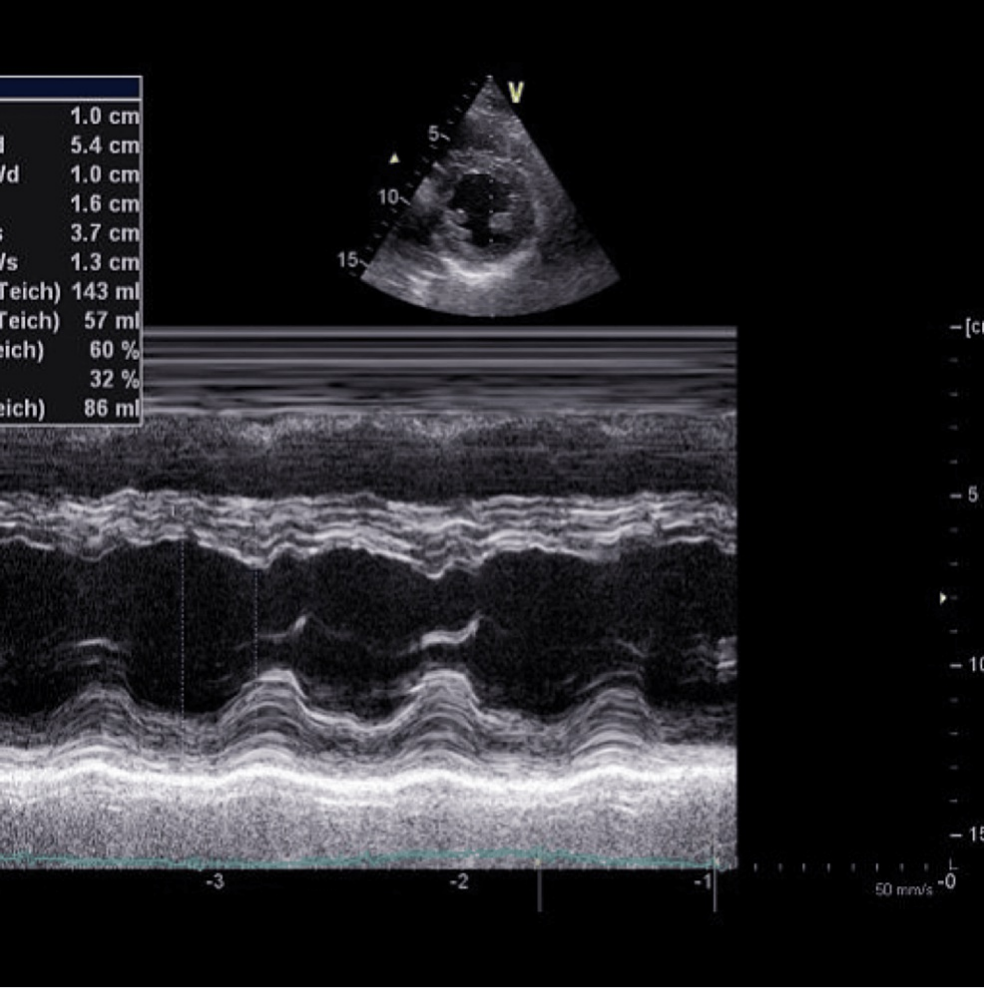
The TTE of the older sibling showed global hypokinesia with reduced LVEF reaching 35%. TTE: transthoracic echocardiogram; LVEF: left ventricular ejection fraction
